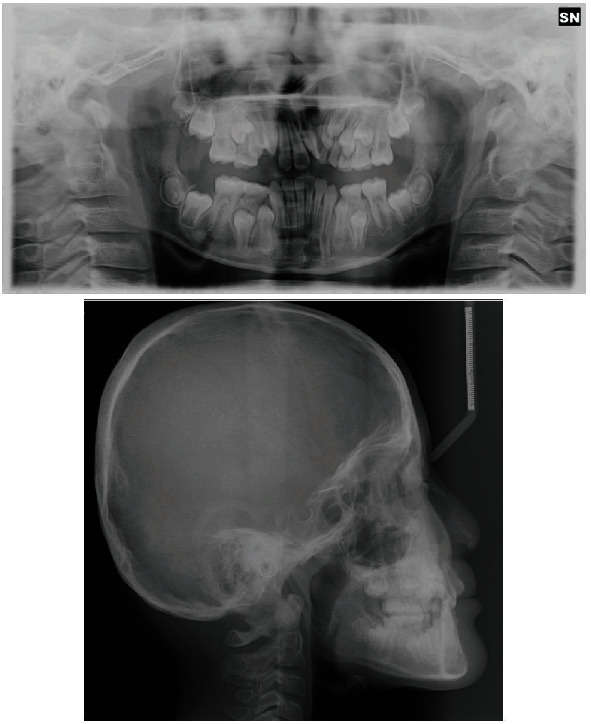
Initial panoramic radiograph and lateral cephalogram of the skull.
